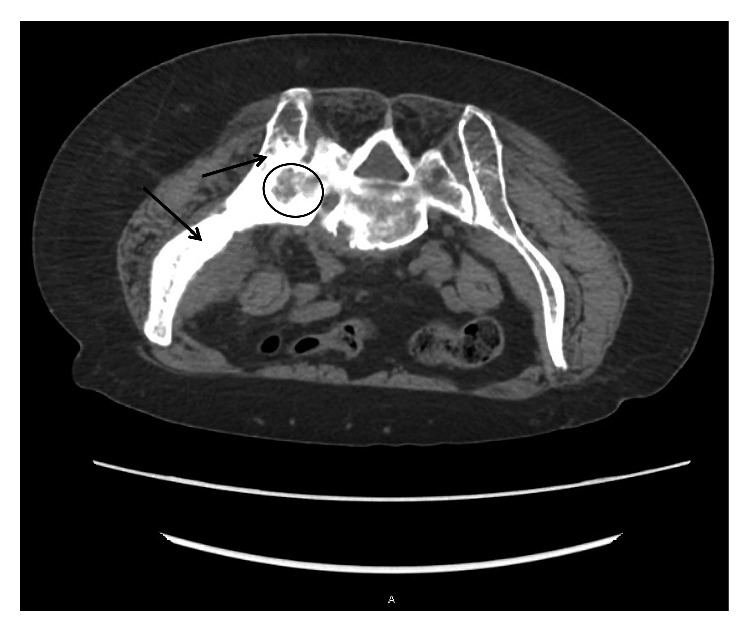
CT biopsy imaging axial body view with slice thickness 5.00 demonstrating lytic (example inside circle) and sclerotic lesions (examples of thickened bone pointed to with arrows) of the left sacrum and left ileum. Also, notice fusion of the left sacro-iliac joint. For reference, the patient is prone in this image as it was taken during the time of biopsy; therefore, the left ileum/sacrum is on the left of the image.
